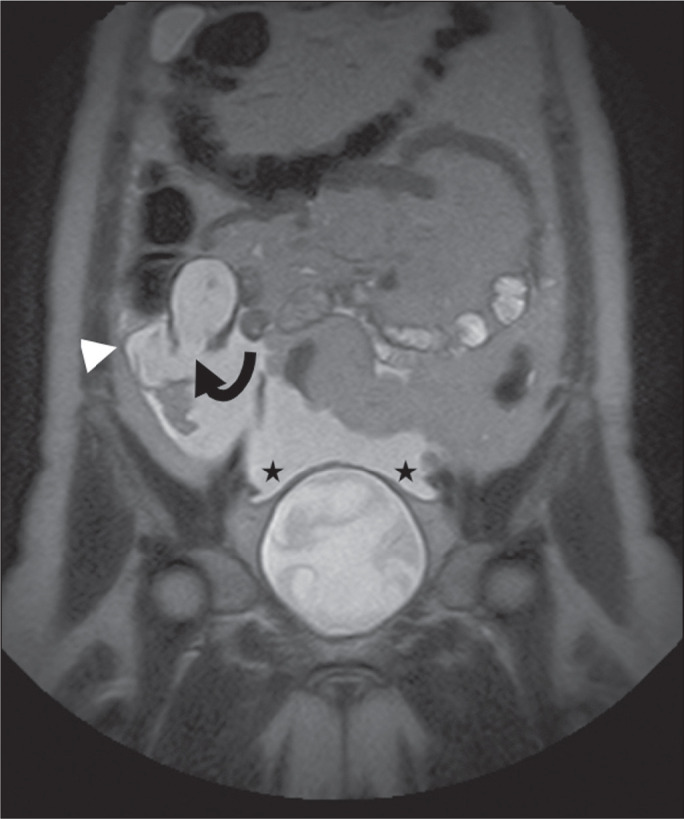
Mucocele of the appendix. Coronal T2-weighted MRI sequence showing a
